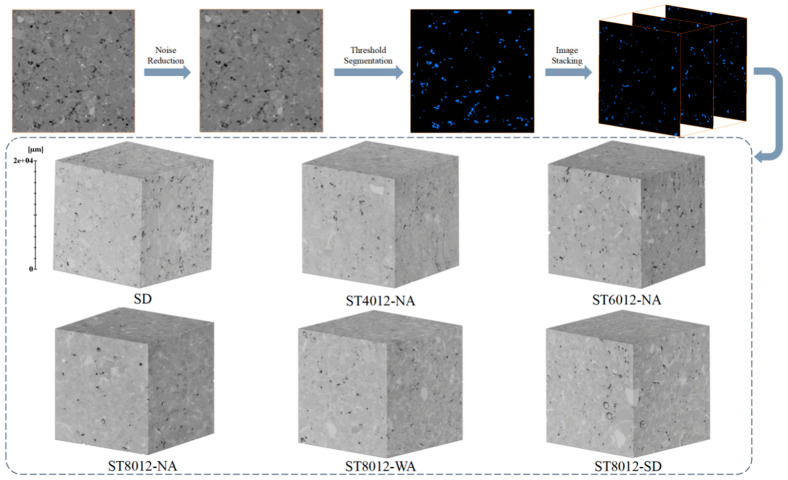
CT image analysis and reconstruction process.
